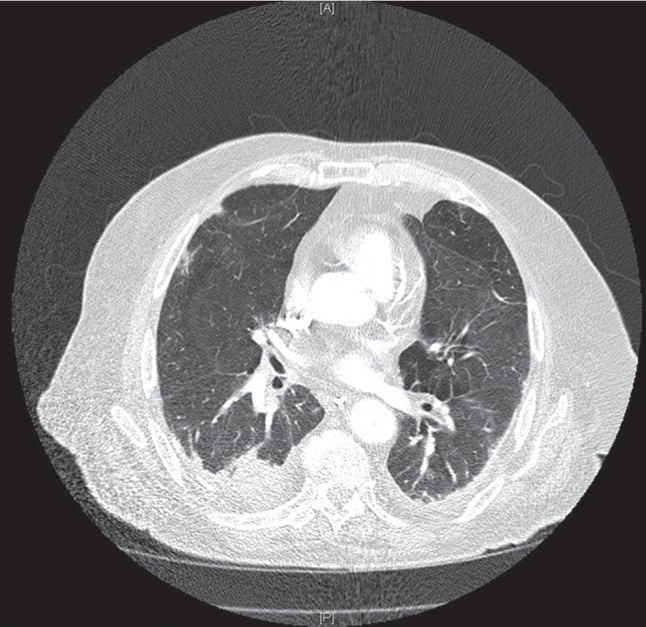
An axial CT chest image of patient 1 demonstrating long-standing bilateral fibrosis and nodular opacities with honey combing and traction bronchiectasis affecting the left lung.
